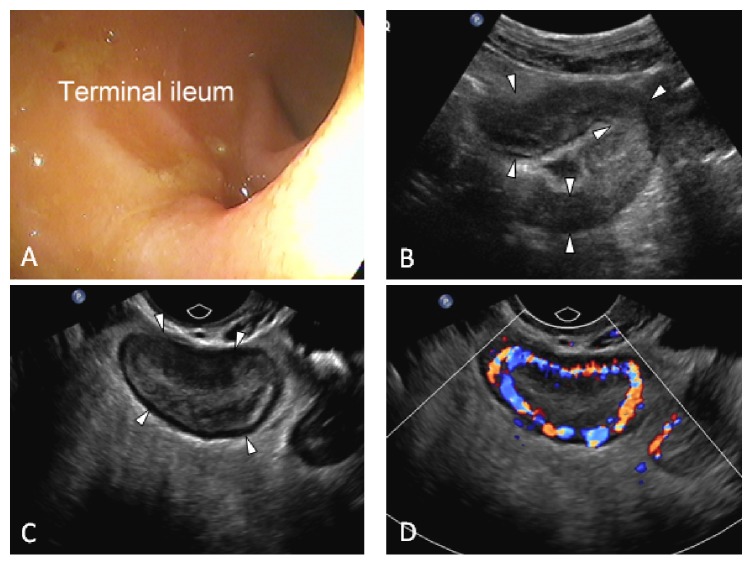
Negative ileocolonoscopy. 26-year-old female with 10-year history of Crohn's disease. Ileocolonoscopy shown in (A) is normal. (B) Transabdominal ultrasound shows a proximal angulated and thickened loop of ileum (arrowheads). (C) is an endovaginal ultrasound scan showing bowel wall thickening and abundant inflammatory fat with superior resolution. In (D) the addition of colour Doppler shows profuse mural vascularity. Interpretation: severe acute inflammation of a proximal ileal loop. Systemic steroids were initiated after ultrasound.
